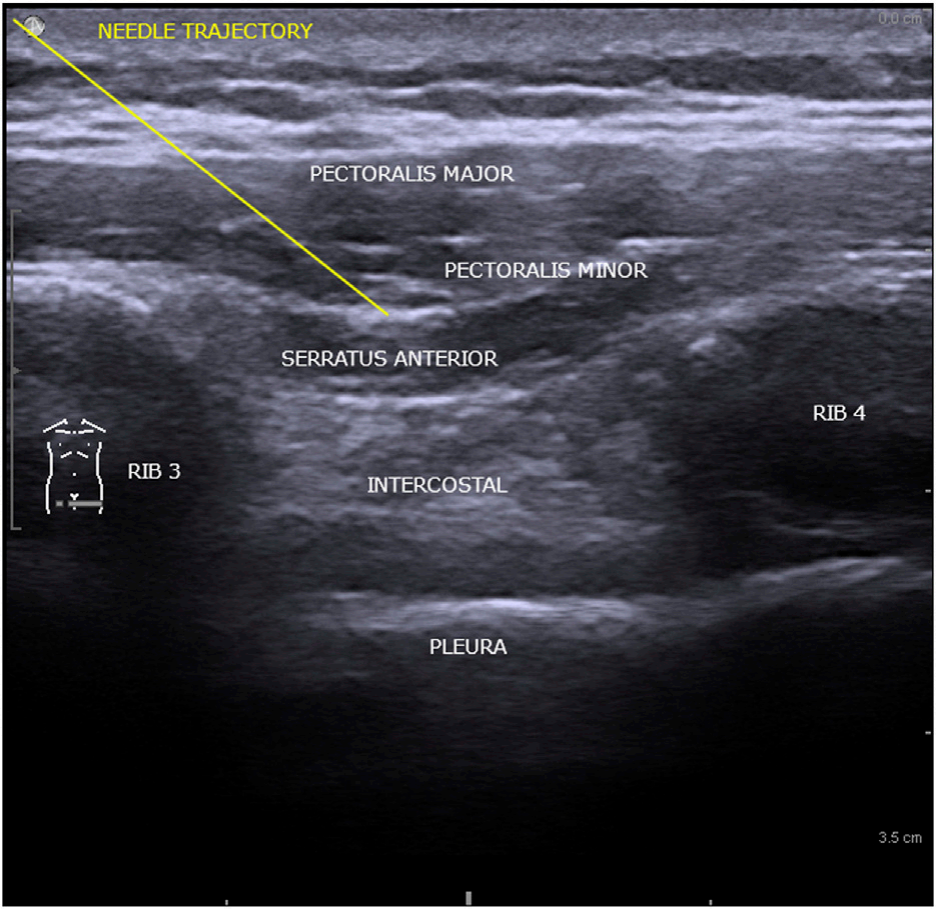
Ultrasonographic structures comprised in interpectoral and pectoserratus plane block.
